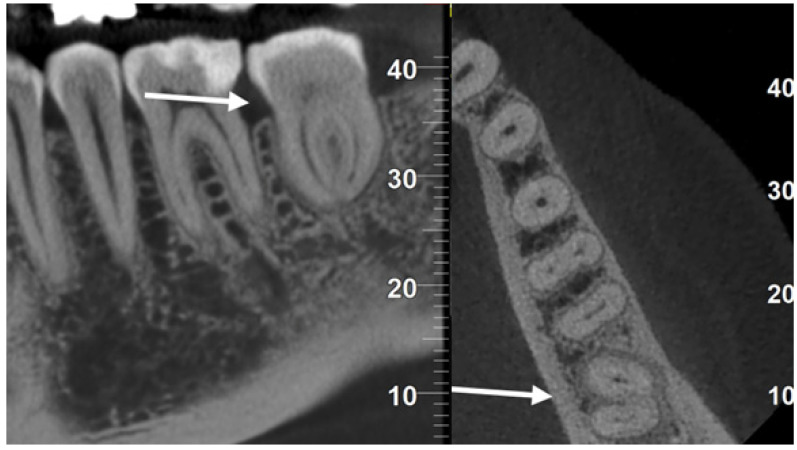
CBCT images at five years showed complete healing even though the transplanted donor tooth (arrows) was not endodontically treated.
